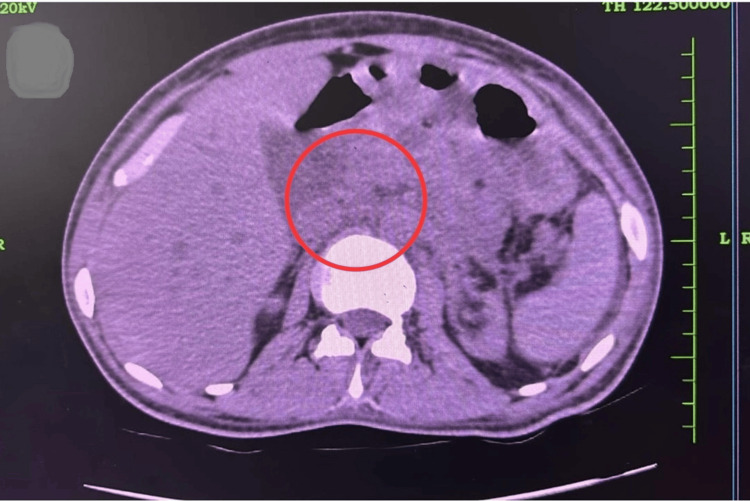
Computed tomography (CT) scan of the abdomen showing an edematous uncinate process and proximal pancreas with fat stranding.
